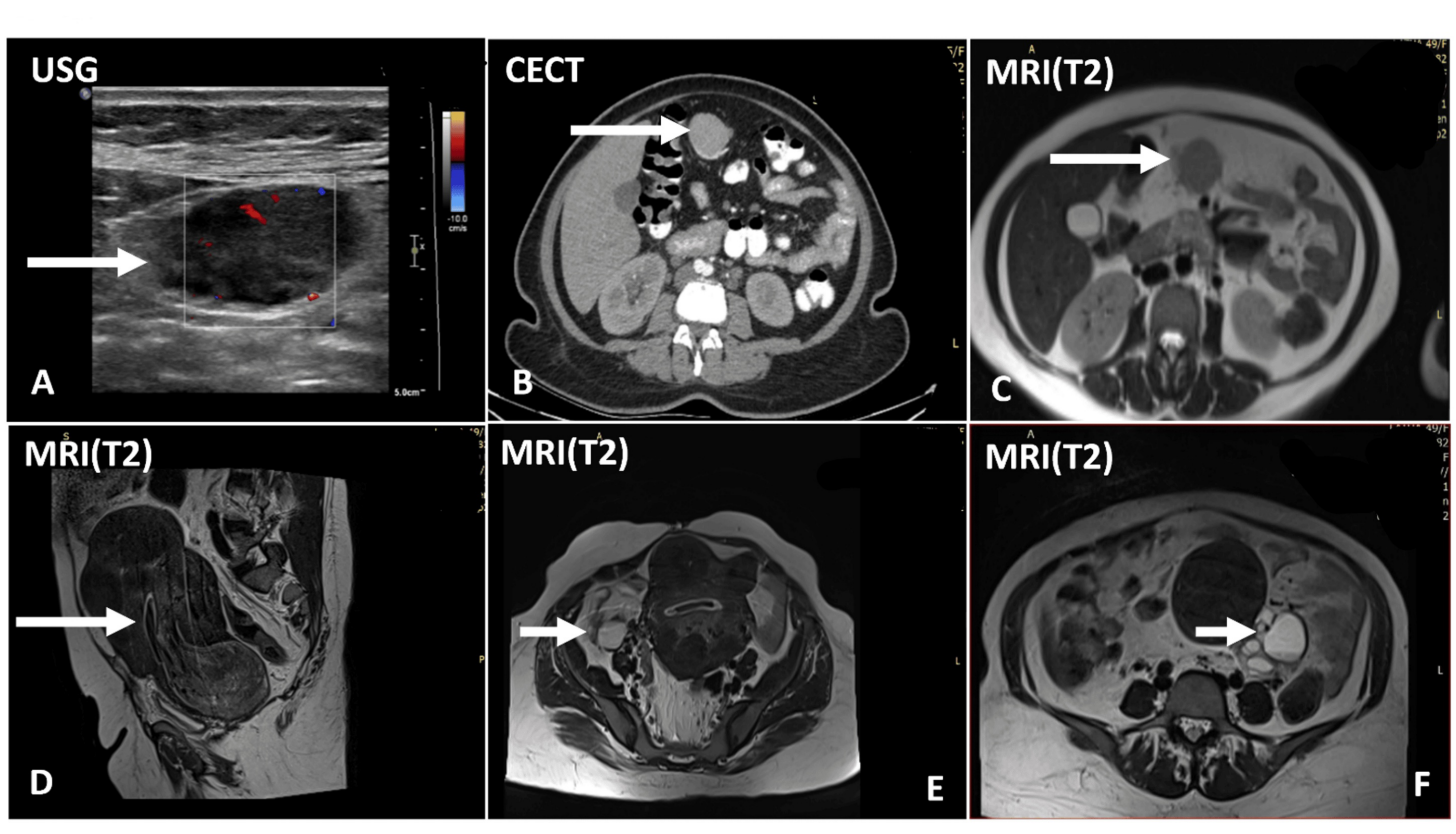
USG of the abdomen (A) shows a relatively well-defined hypoechoic lesion with areas of internal vascularity in the epigastric region (box in A). CECT of the abdomen (B) shows a homogeneously enhancing soft tissue density lesion in the epigastric region (arrow in B). MRI of the abdomen (C) shows a T2 isointense lesion in the epigastric region (arrow in C), a large submucosal fibroid (arrow in D), a right ovarian cyst (arrow in E), and a left ovarian cyst (arrow in F) USG: ultrasonogram; CECT: contrast enhanced computed tomography; MRI: magnetic resonance imaging
